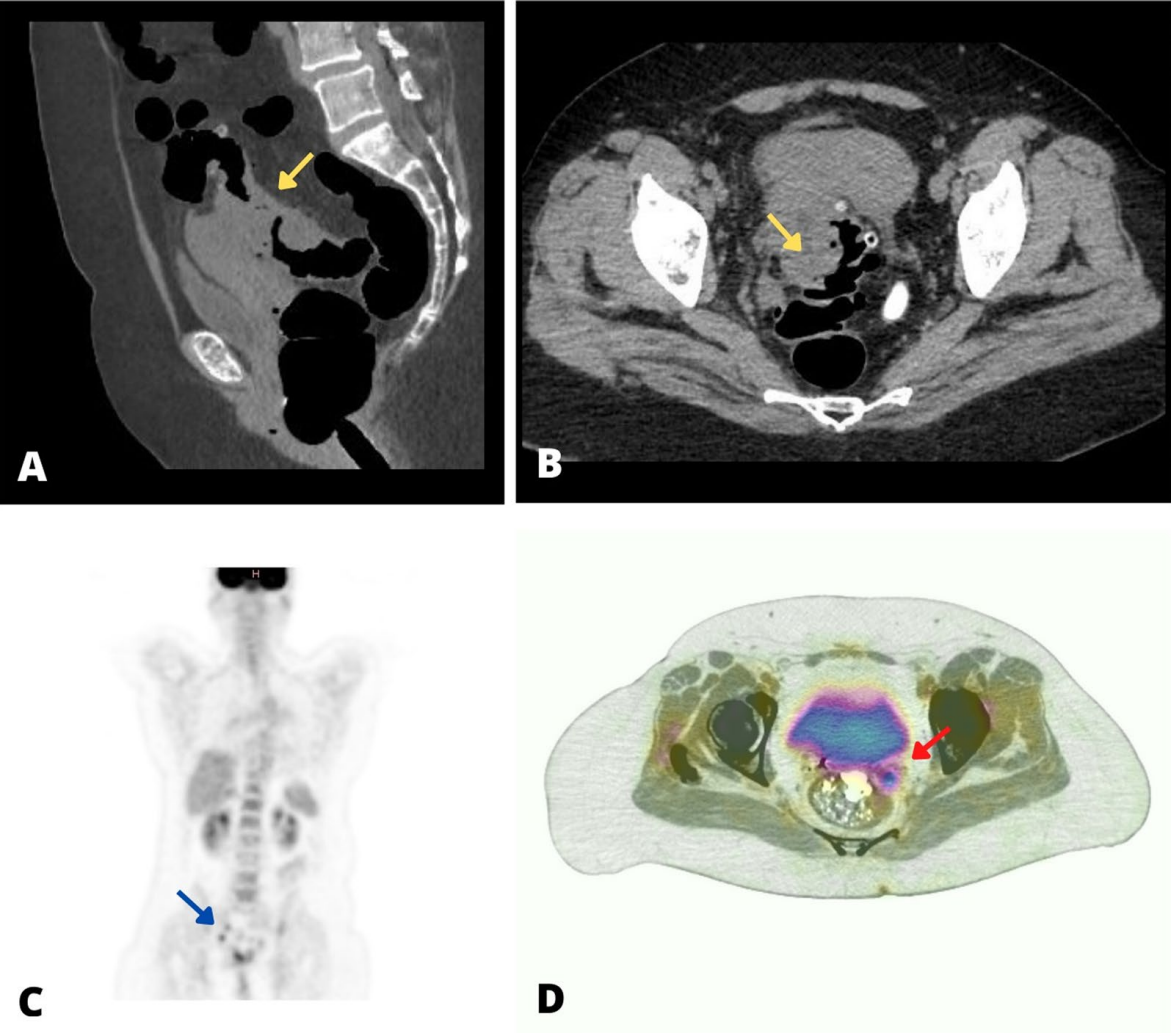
Advanced Imaging. Panels A and B are computed tomography showing the primary lesion involving the uterus and lumen of the colon (yellow arrow). Panel C is the first Positron Emission Tomography and Computed Tomography (PET-CT) scan showing two hypermetabolic nodules (< 1 cm) near the right lymphatic iliac chain (blue arrow). Panel D is the second PET-CT ordered after the second chemotherapeutic regimen, revealing a hypermetabolic nodular (2 × 2 cm) lesion behind the bladder near the sigmoid colon (red arrow)
